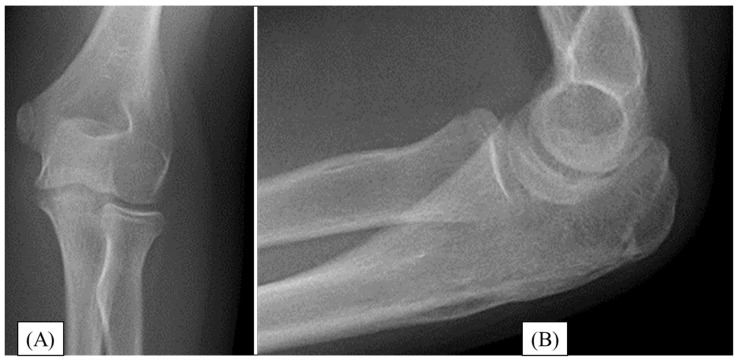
One year after the reconstruction of the olecranon fracture. Control X-rays show perfect articular congruency and a completely healed fracture from AP (A) and lateral views (B).
